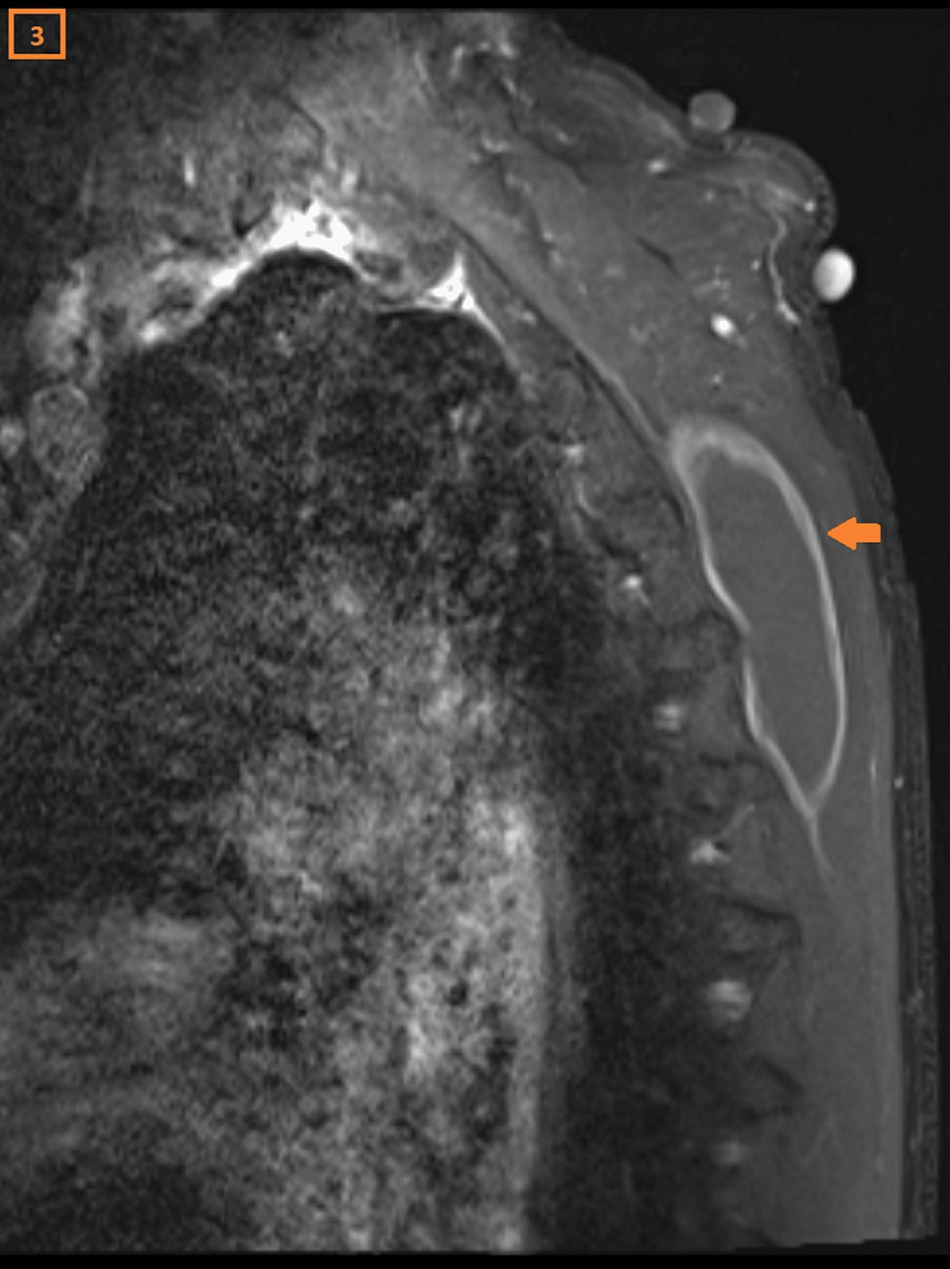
Post-contrast image of cystic lesion The axial T1, sagittal T2, and post-contrast MRI images of the scapulothoracic region show an elongated lesion hyperintense on T2, and isointense on T1 with peripheral contrast enhancement.
